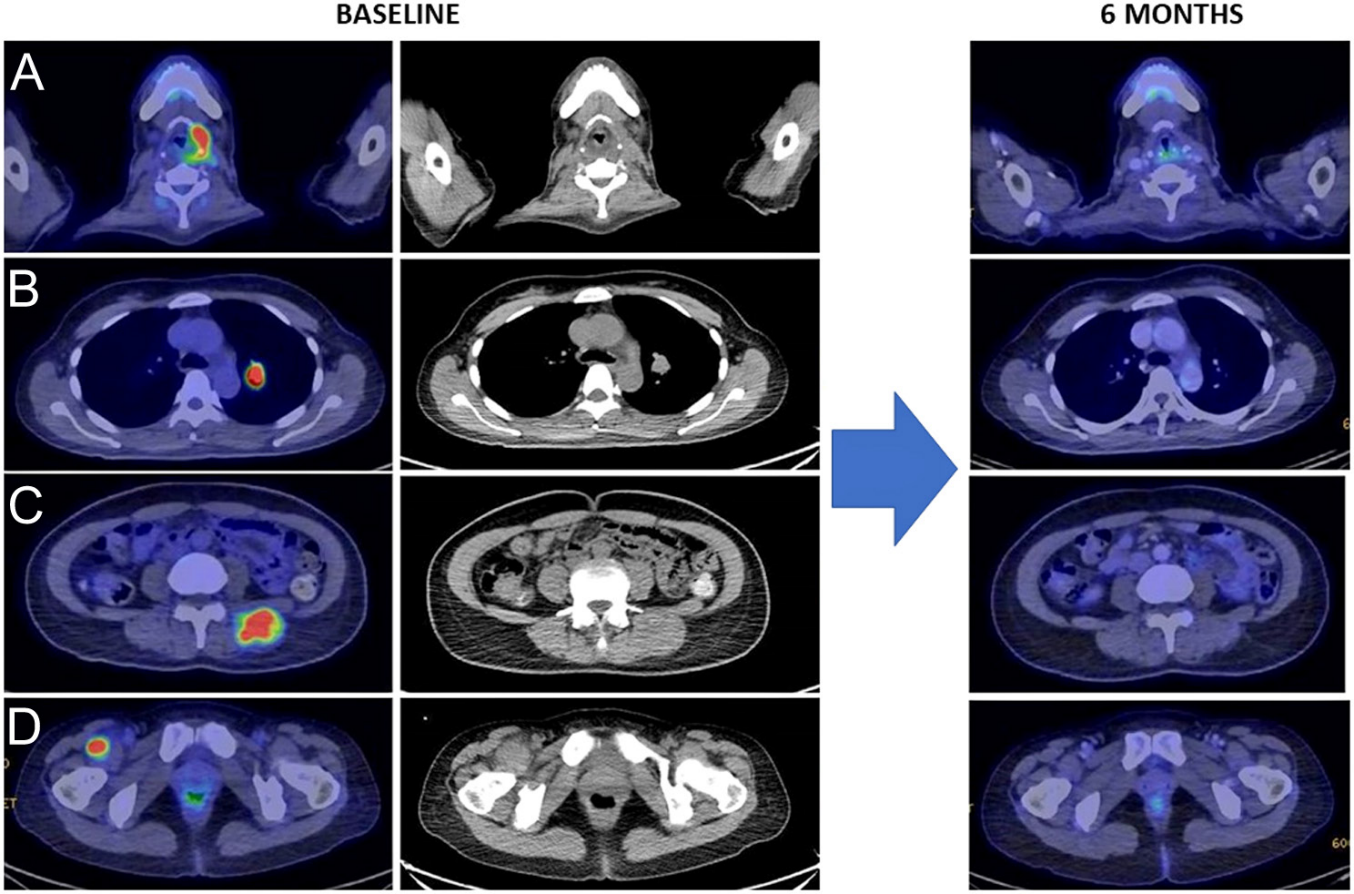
Serial FDG-PET/CT scans at baseline and at the 6-month timepoint from patient 1 showing complete response at both local and distant metastatic sites at 6 months after treatment initiation. A. Thyroid bed. B. Left hilar lymph node. C and D. Muscle.
